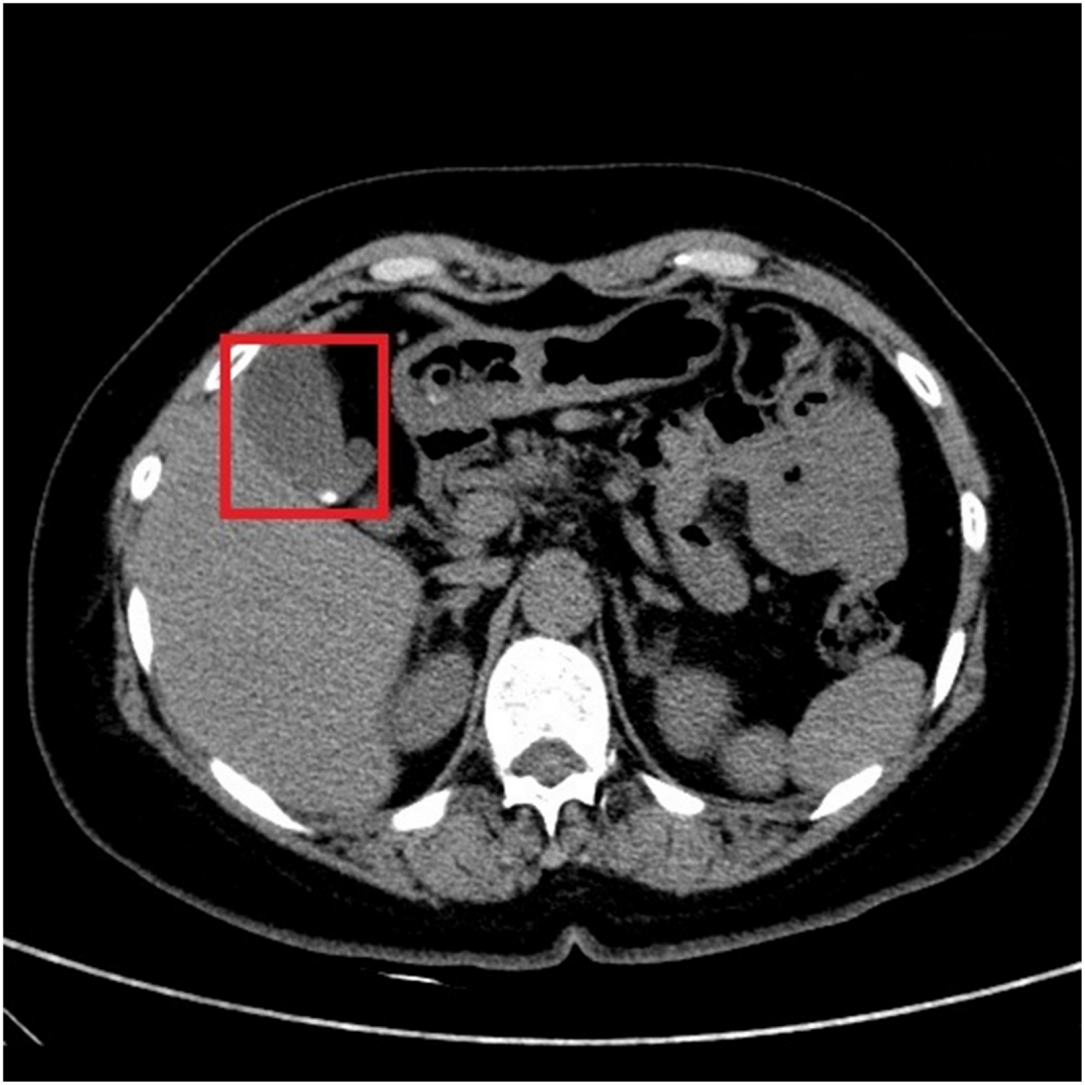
The CT image of a person has a lower degree of disease.
